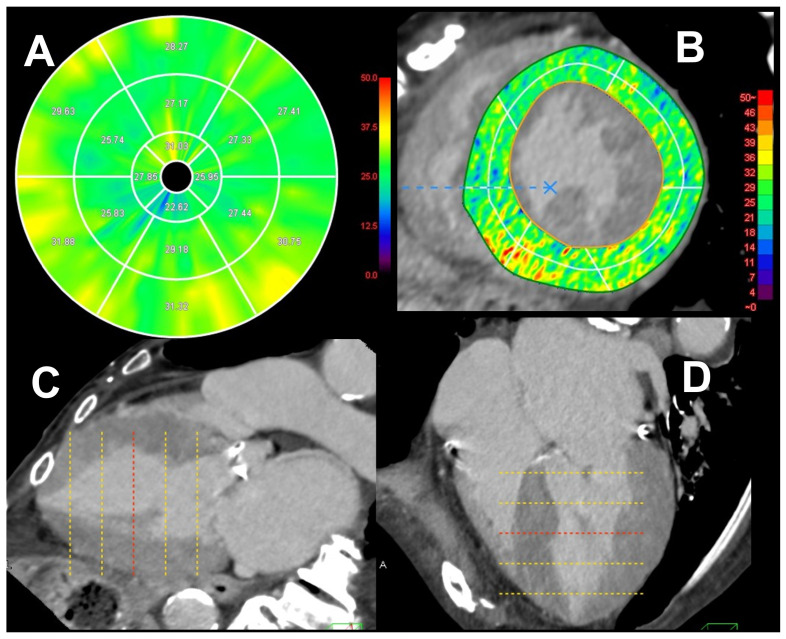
Details for left ventricular extracellular volume analysis in CT. The left ventricular (LV) extracellular volume (ECV) (LV-ECV) measurements were obtained from non-contrast and late-phase cardiac CT scans using commercially available software (Ziostation 2, Ziosoft Inc, Tokyo, Japan). Segmental LV-ECV was analyzed based on the 16-segment model of the LVM (A). ECV analysis was performed using short-axis images and two- and four-chamber views of the late-phase cardiac CT images (B–D). The yellow dotted line in the LV long-axis image shows the short-axis slice for analysis, while the image presented in the top right corner shows the cut plane of the red dotted line.
